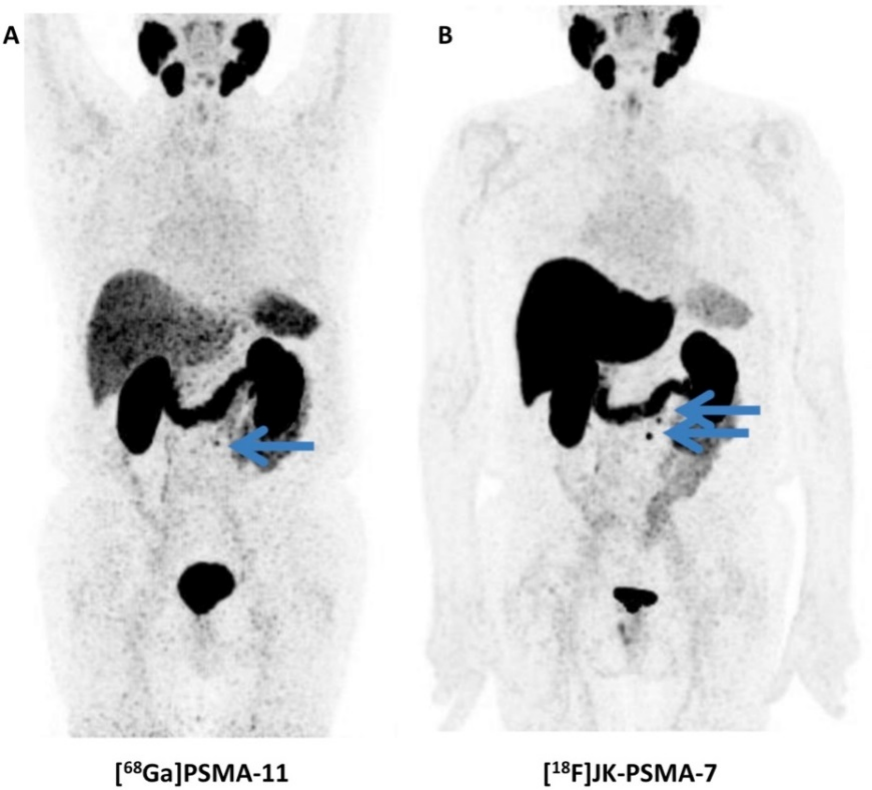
Head-to-head comparison of [^68^Ga]PSMA-11 vs. [^18^F]JK-PSMA-7. Whole-body distribution of [^68^Ga]PSMA-11 (A) vs. [^18^F]JK-PSMA-7 (B) in the same patient. [^68^Ga]PSMA-11 revealed only one PSMA-positive retroperitoneal paraaortal lymph node (blue arrow), whereas the [^18^F]JK-PSMA-7 PET/CT showed two PSMA-positive retroperitoneal lymph nodes (blue arrows). Modified from *Dietlein* et al. 109, © by the Society of Nuclear Medicine and Molecular Imaging, Inc.
